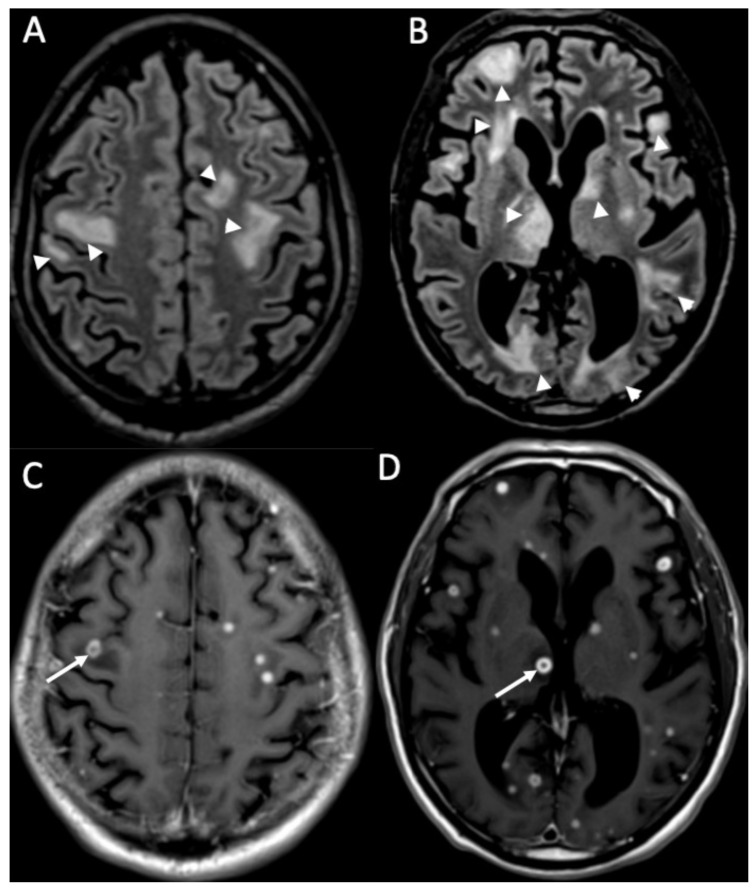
Axial FLAIR (A,B) and enhanced T1 (C,D) depict multiple tiny foci of enhancement, some of which have a ring appearance (arrows in C,D) in a patient with miliary tuberculosis. They are surrounded by vasogenic edema (arrowheads in A,B).
